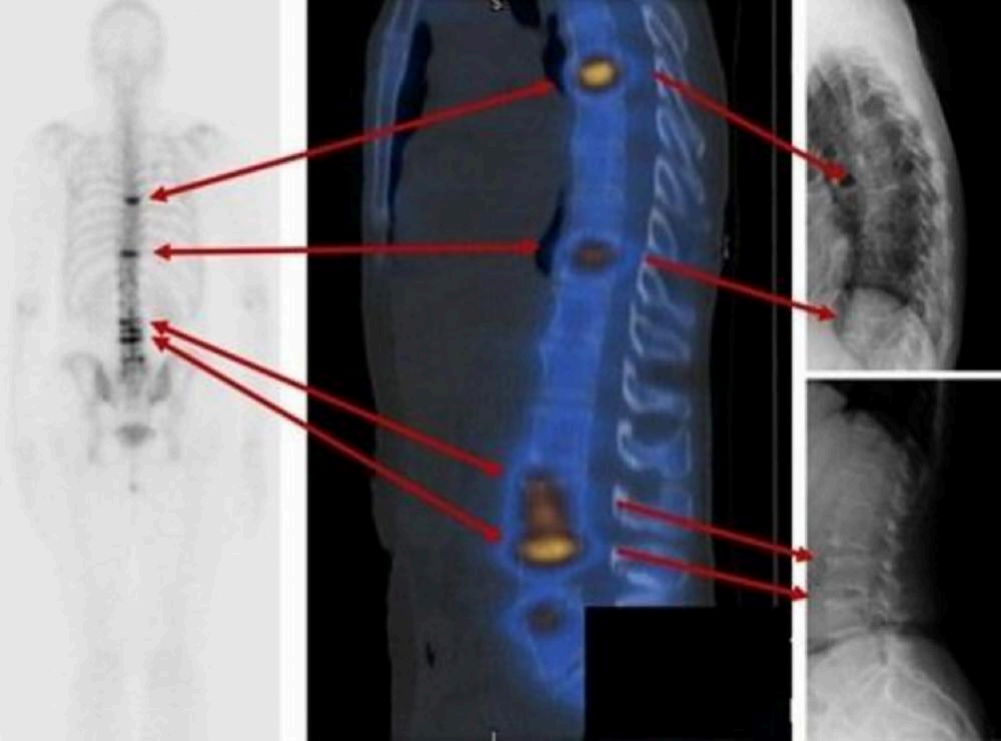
Nuclear medicine bone scan with single-photon emission computed tomography/computed tomography imaging and lateral spine X-ray. Horizontal bands of increased activity at T7, T11, L3, and L4 due to acute fractures.
